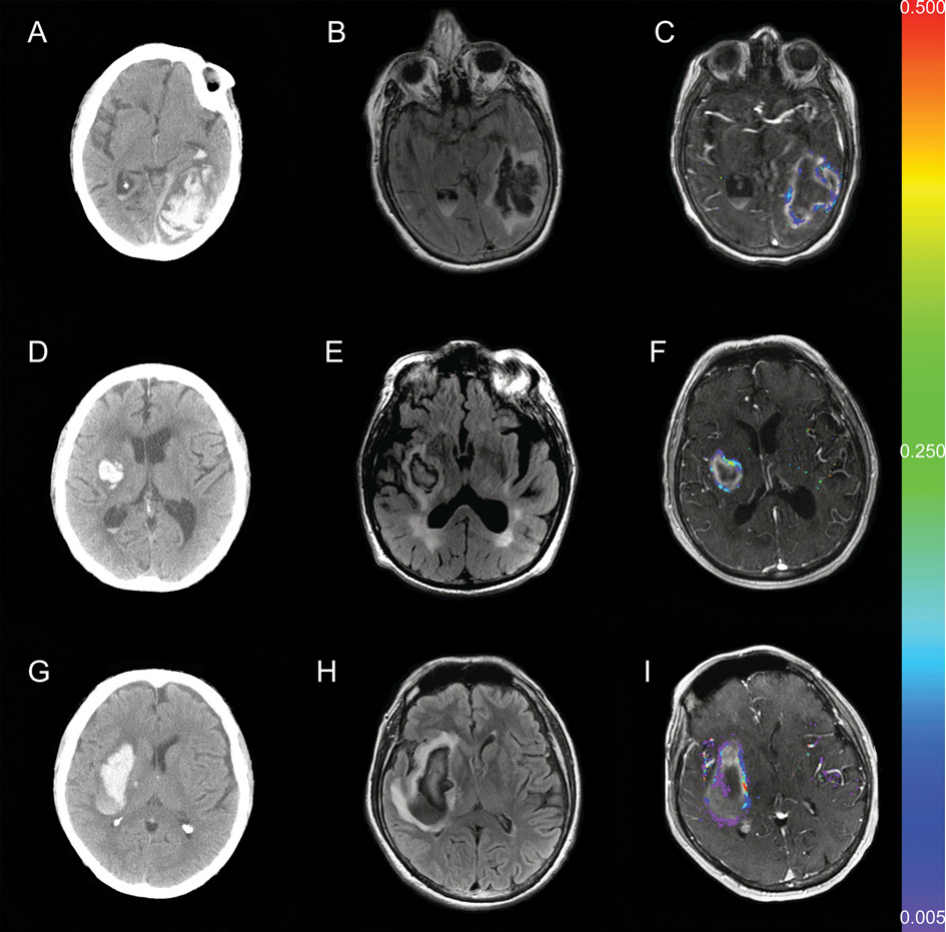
Admission brain CT, 1‐day FLAIR MRI and 1 week color coded permeability maps of a patient with a lobar hematoma (A, B, C), a patient with a small basal ganglionic (deep) hematoma (D, E, F), and a patient with a large basal ganglionic (deep) hematoma (G, H, I). BBB disruption around the hematoma at 1 week after symptom onset is readily visible on the permeability maps in all 3 patients. Color coded K^trans^ values are as described in the legend of Figure 2. CT indicates computed tomography; FLAIR, fluid attenuated inversion recovery; MRI, magnetic resonance imaging; BBB, blood–brain barrier.
